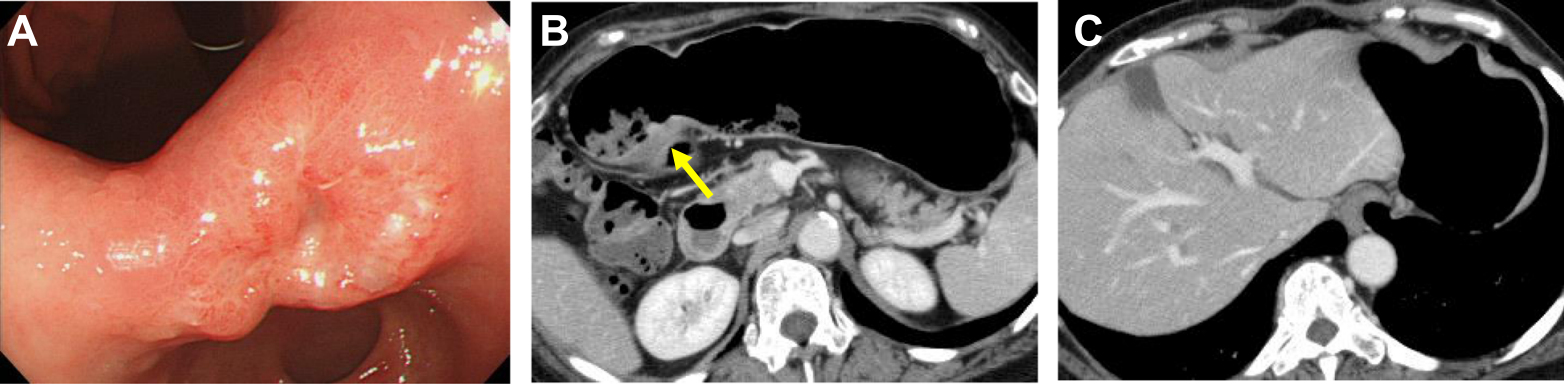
a Esophagogastroduodenoscopy revealed an irregular and nodular tumor on the middle third region of the stomach. b Computed tomography (CT) showed thickening of the gastric wall in tumor site (arrow). c No lymph node metastasis or distant metastasis was detected by CT
